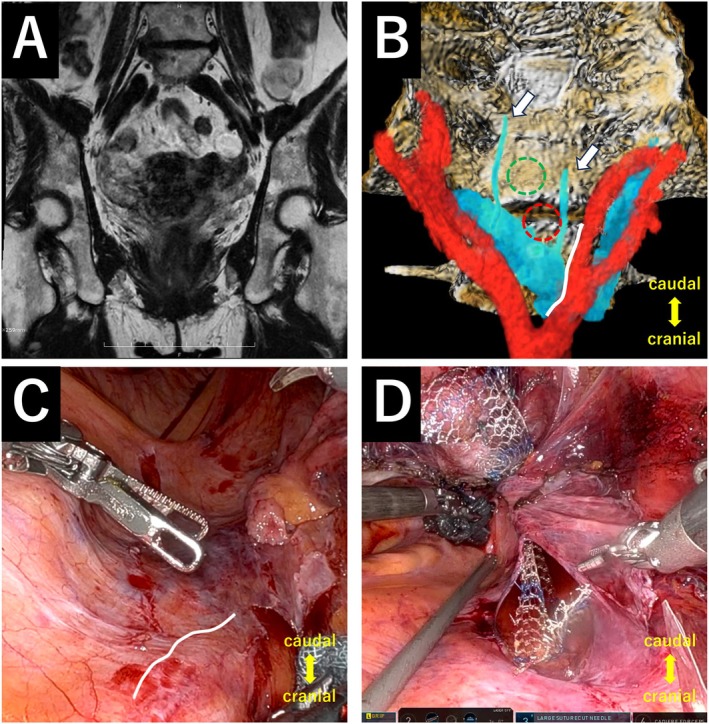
Preoperative three‐dimensional (3D) reconstructed imaging and intraoperative navigation in Case 1. (A) Coronal T2‐weighted magnetic resonance imaging. (B) Preoperative 3D reconstructed imaging generated using the SYNAPSE VINCENT system demonstrating vascular anatomy around the sacral promontory. Arterial structures are shown in red and venous structures in blue. The yellow circle indicates the narrow vascular window identified on preoperative 3D reconstruction. The green circle indicates the selected fixation site on the anterior surface of S1. White arrows indicate venous branches running along the anterior sacral surface, which were also considered during preoperative surgical planning. The white line indicates the medial border of the right common iliac artery continuing to the internal iliac artery. (C) Intraoperative view during sacral promontory dissection. The right common iliac artery was identified by arterial pulsation and gentle palpation using the robotic forceps. This vessel served as the anatomical landmark for correlating the preoperative 3D reconstructed image with the operative field. (D) Intraoperative findings during robot‐assisted sacrocolpopexy with mesh fixation on the anterior longitudinal ligament over S1.
